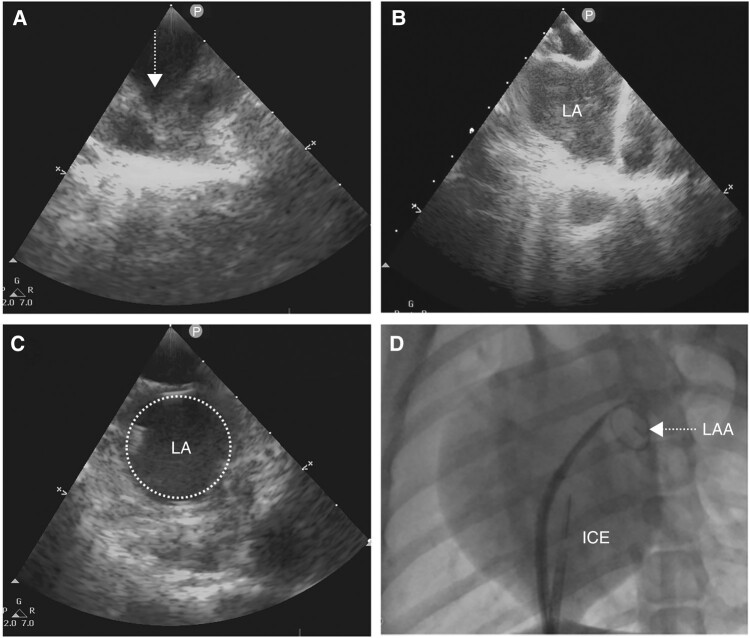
The atrial septal puncture under the guidance of ICE. (*A*) The ICE image showed RA, LA, and atrial septum, and the puncture sheath delivered forward against the atrial septum to form the ‘tent sign’. (*B*) Puncture the needle into the LA smoothly; saline injection can be seen ‘bubble sign’. (*C*) The steel wire was sent along the sheath tube and coiled; the LA was full of ‘ring’. (*D*) The circular guidewire was located in the LAA under fluoroscopy. ICE, intracardiac echocardiography; RA, right atrial; LA, left atrial; LAA, left atrial appendage.
